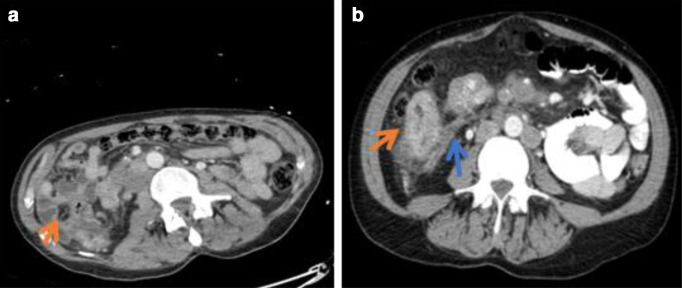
(a) Axial contrast-enhanced CT abdomen showing normal non-dilated bowel loops in the lower quadrant at the level of the caecum (red arrow). (b) Axial contrast-enhanced CT abdomen performed 4 months after (a) shows significant thickening of the caecum (red arrow) with circumferential luminal narrowing and extensive surrounding fat stranding and mesenteric invasion along the medial aspect of the caecal mass (blue arrow).
